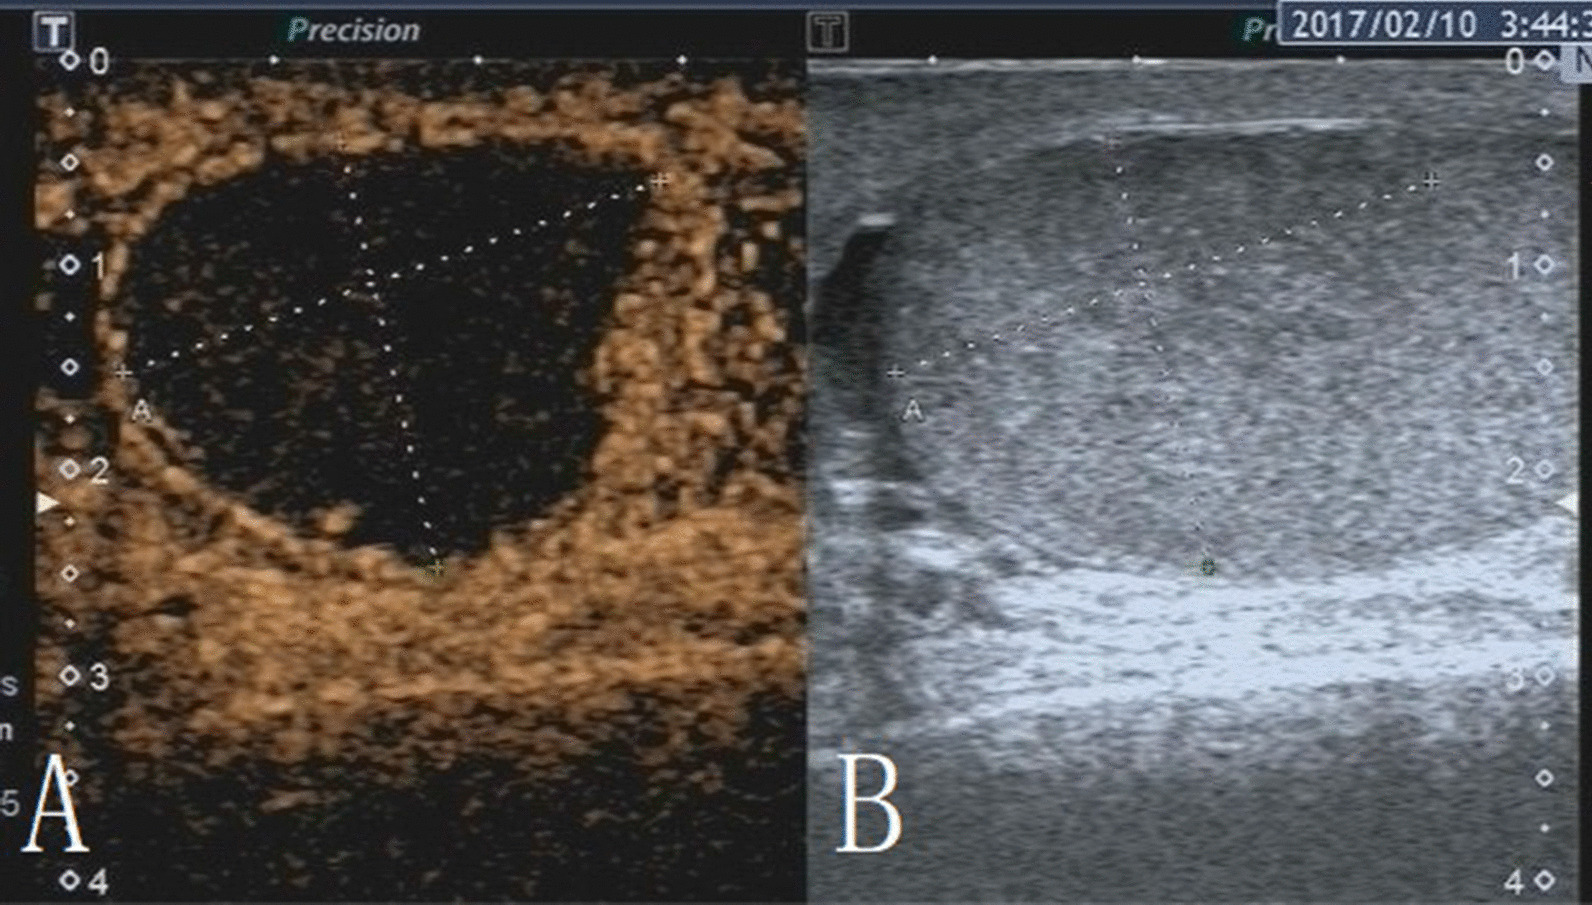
Testicular segmental infarction. A Contrast-enhanced ultrasonography showed no enhancement; the diagnosis was segmental infarction. B Conventional ultrasonography showed ill-defined iso-echo signals in the middle and upper part of the left testis. It was impossible to determine whether this was a tumor or not
